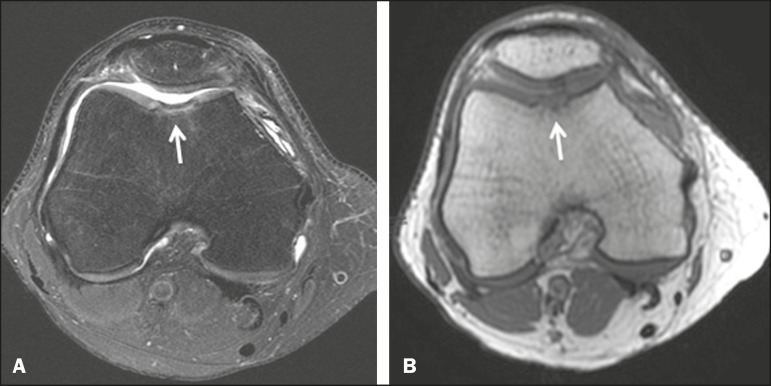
A 31-year-old man submitted to the biomembrane procedure. Postoperative axial T2-weighted fat-saturated MRI sequence (A) showing patellar chondropathy characterized by sparse foci of altered signal intensity, slight narrowing with superficial irregularities in the medial aspect of the vertex and deep fissure in its middle third, accompanied by mild subchondral bone edema. Signs of chondroplasty in the sulcus (arrow) and beginning of the aspects of the femoral trochlea, with a thin collagen membrane and narrow, irregular fibrocartilaginous tissue covering the subchondral bone, which is also irregular, with foci of edema. A T1-weighted sequence acquired at two months after the procedure (B), showing the area where the membrane joins the adjacent cartilage, where there is a slight change in signal intensity (arrow).
